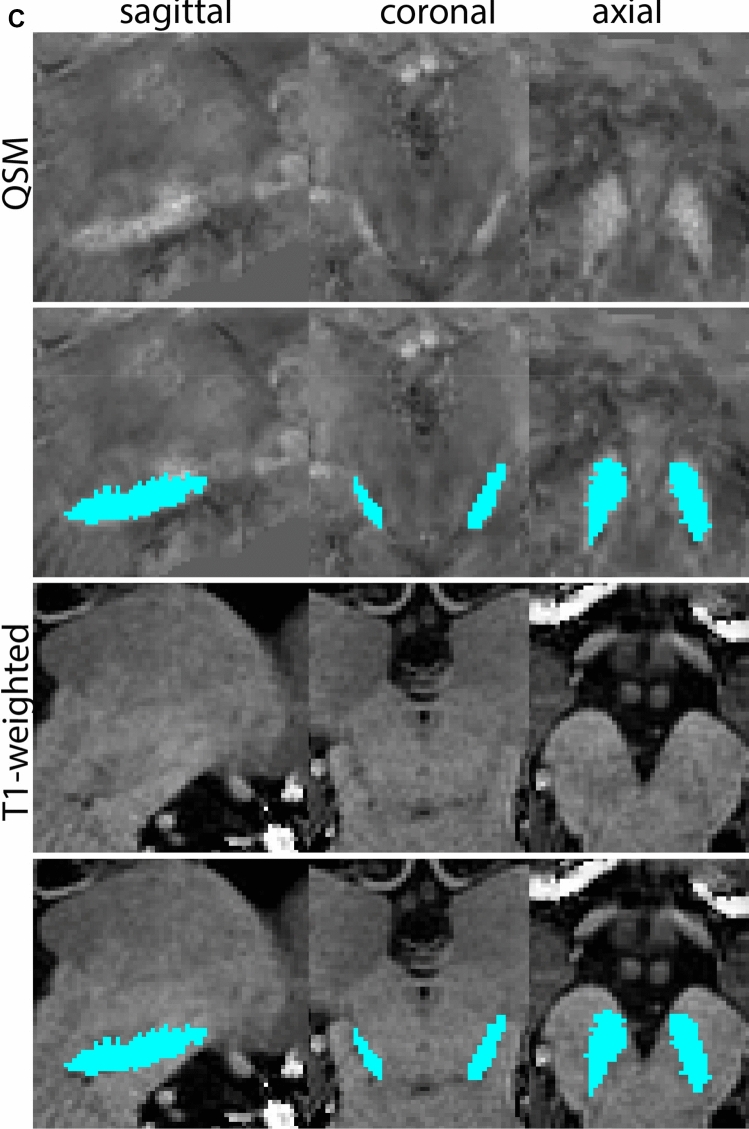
a The substantia nigra (SN) appears as a hyperintense structure on the QSM contrast as a result of its high iron content. In coronal levels, the medial part of the SN lies inferior to the lateral parts of the SN. The RN provides a clear anatomical reference point. Note the limited visibility of the SN on the T1-weighted contrast. b The substantia nigra (SN) decreases in size in more rostral levels. Note the clear visibility of the mamillary bodies at these levels in the T1-weighted contrast. c The substantia nigra (SN) decreases in size and appears in a larger angle in more caudal levels
